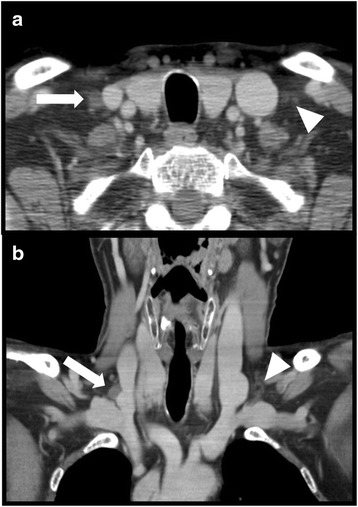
A 55 year old male with laryngeal carcinoma. Contrast enhanced computed tomography in the axial (a) and coronal (b) plane. Sacciform configuration of the right lymphatic duct in both planes (arrows). The thoracic duct shows a dendritic morphology (arrowheads)
